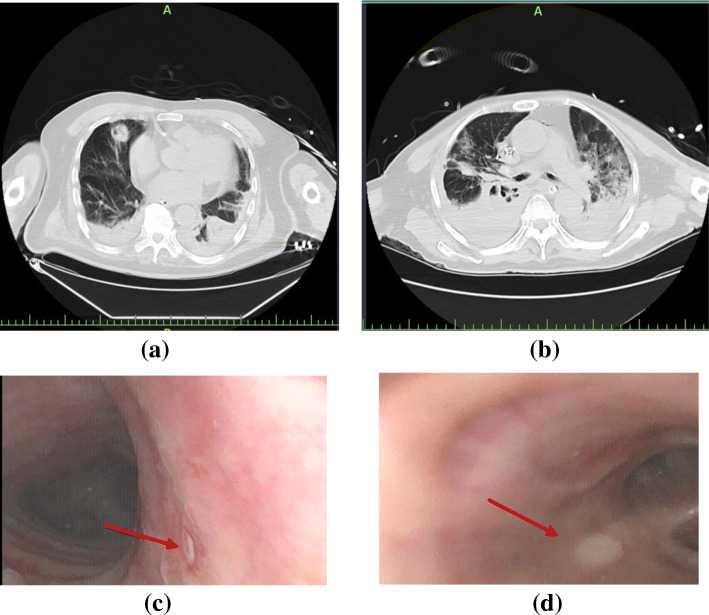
Lung CT scans and bronchoscopy findings of patients with COVID-2019 and IPA. Patient 1, 90 years, lung CT scan showed nodules with cavities in the middle lobe of the right lung and consolidation bilateral lower lobes with a small amount of pleural effusion (a); bronchoscopy finding showed small ulcer in the right wall of the trachea (c). Patient 2, 74 years, lung CT scan showed consolidation bilateral lower lobes with multiple irregular cavities in the middle (b); bronchoscopy finding showed right middle bronchial ulcer (d). Red arrow indicated the positions of bronchial ulcers
